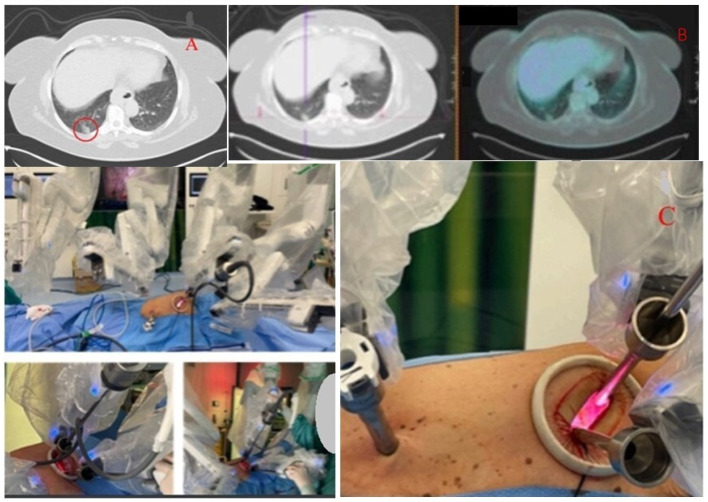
Preoperative CT, showing pulmonary lesion in red circle (A) and PET (B). Surgical port mapping (C).
